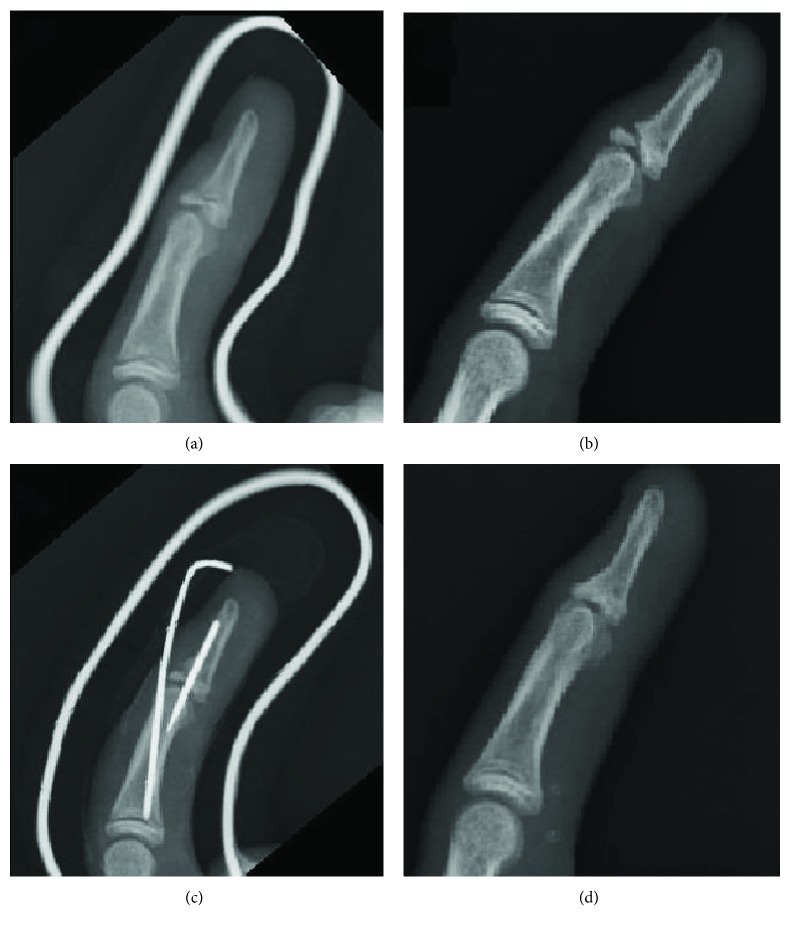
Case 6: lateral view radiographs of a 12-year-old boy. (a) Preoperative radiograph at 12 weeks after injury. (b) Bone union was not achieved after conservative treatment with an aluminum splint. (c) Postoperative radiograph at 30 weeks after injury. (d) The Crawford score was excellent at 50 weeks after the surgery.
